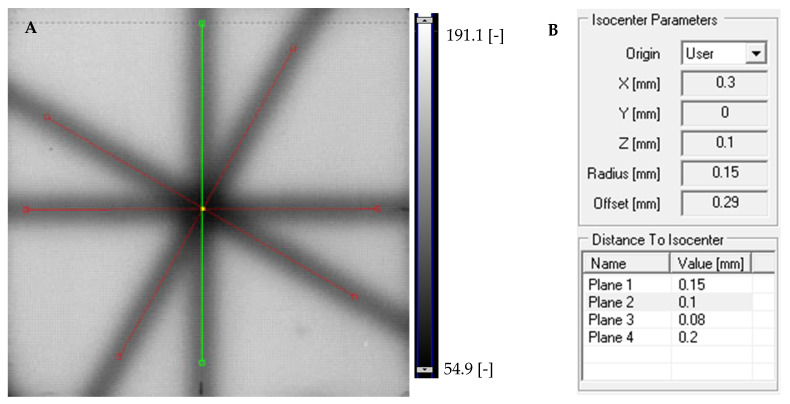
Application of Fricke-XO-Gelatine with sorbitol to the coincidence test of radiation and mechanical isocenters for the TrueBeam (Varian, Las Vegas, NV, USA) medical accelerator. (A): Green channel image obtained by splitting the sample scan into RGB color model channels viewed in the polyGeVero-CT software package (GeVero Co., Poland). Red and green lines are specific tools for determining the isocenter, while the yellow dot indicates the location of the radiation isocenter. (B): Results of the test, isocenter parameters: location, radius, and offset (distance of the radiation isocenter to the mechanical isocenter of the medical accelerator).
